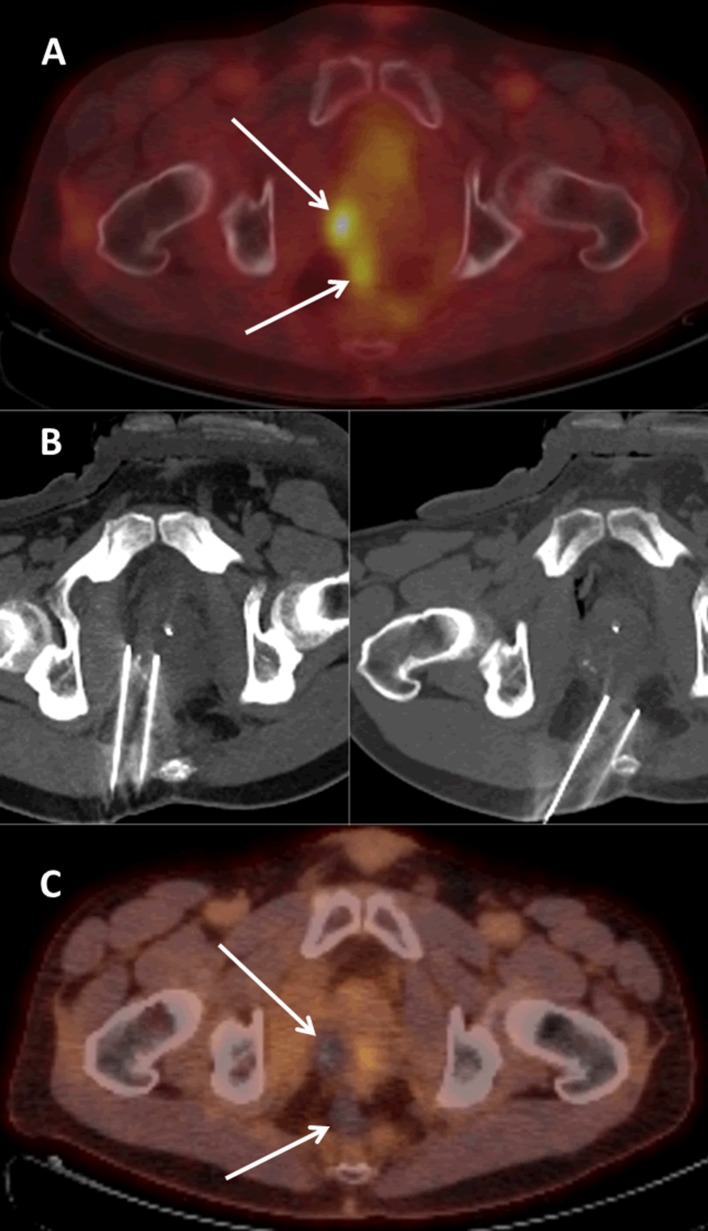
18F-FDG PET-CT image of a 48-year-old male patient with two small pathologically proven locoregional recurrences (*arrows*) of primary rectal adenocarcinoma in the precoccygeal and right peri-prostatic area (A). Nonenhanced CT scan showing the inserted needle electrodes prior to pulse delivery (B). 18F-FDG PET-CT image 3 months after IRE showing no signs for residual or recurring disease (C)
